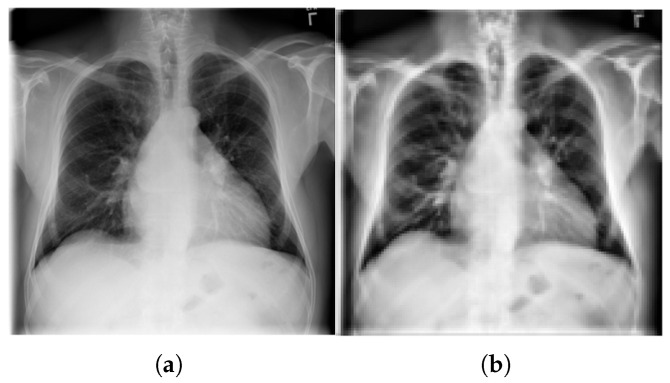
Comparison between (a) the original grayscale chest X-ray image at a resolution of 1024×1024 pixels, and (b) the image after preprocessing using CLAHE and rescaling to 128×128 pixels.
